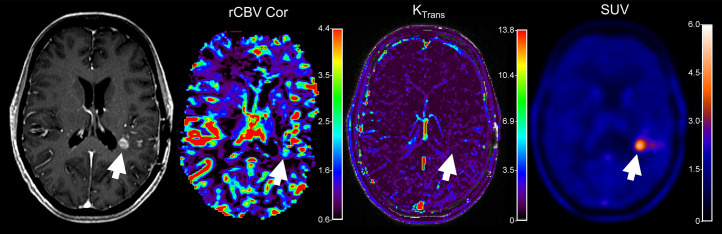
Patient with suspected recurrence of left parietal glioma (arrows) of World Health Organization central nervous system grade 4. Patient was initially evaluated with ^18^F-FET PET/MRI for increasing contrast-enhanced T1-weighted MRI findings posterior to left resection cavity. Dynamic contrast-enhanced K_trans_ parameter met threshold for progression (0.38), but maximum rCBV value remained borderline (3.0). ^18^F-FET PET TBR_max_ and TBR_mean_ exceeded threshold at 2.75 and 2.3, respectively, supporting diagnosis of disease progression. Bevacizumab treatment was initiated, and enhancement and perfusion pattern improved on follow-up MRI, remaining stable for 2.5 y. Serial ^18^F-FET PET/MRI 2 y later remained stable.
